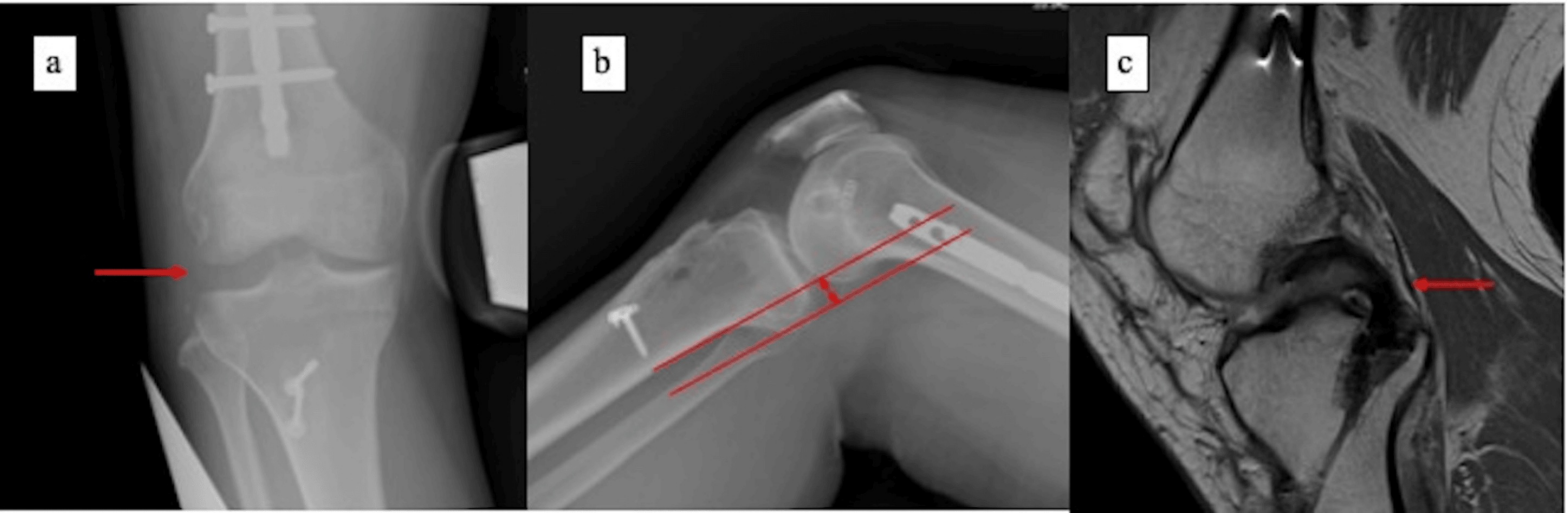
Final imaging findings (a) Varus stress radiographs at final follow-up showing improved lateral stability compared with the preoperative status. (b) Gravity-sag views demonstrating an approximately 3-mm side-to-side difference between the affected and unaffected knees. (c) MRI at final follow-up confirming good graft/ligament continuity of the posterior cruciate ligament.
